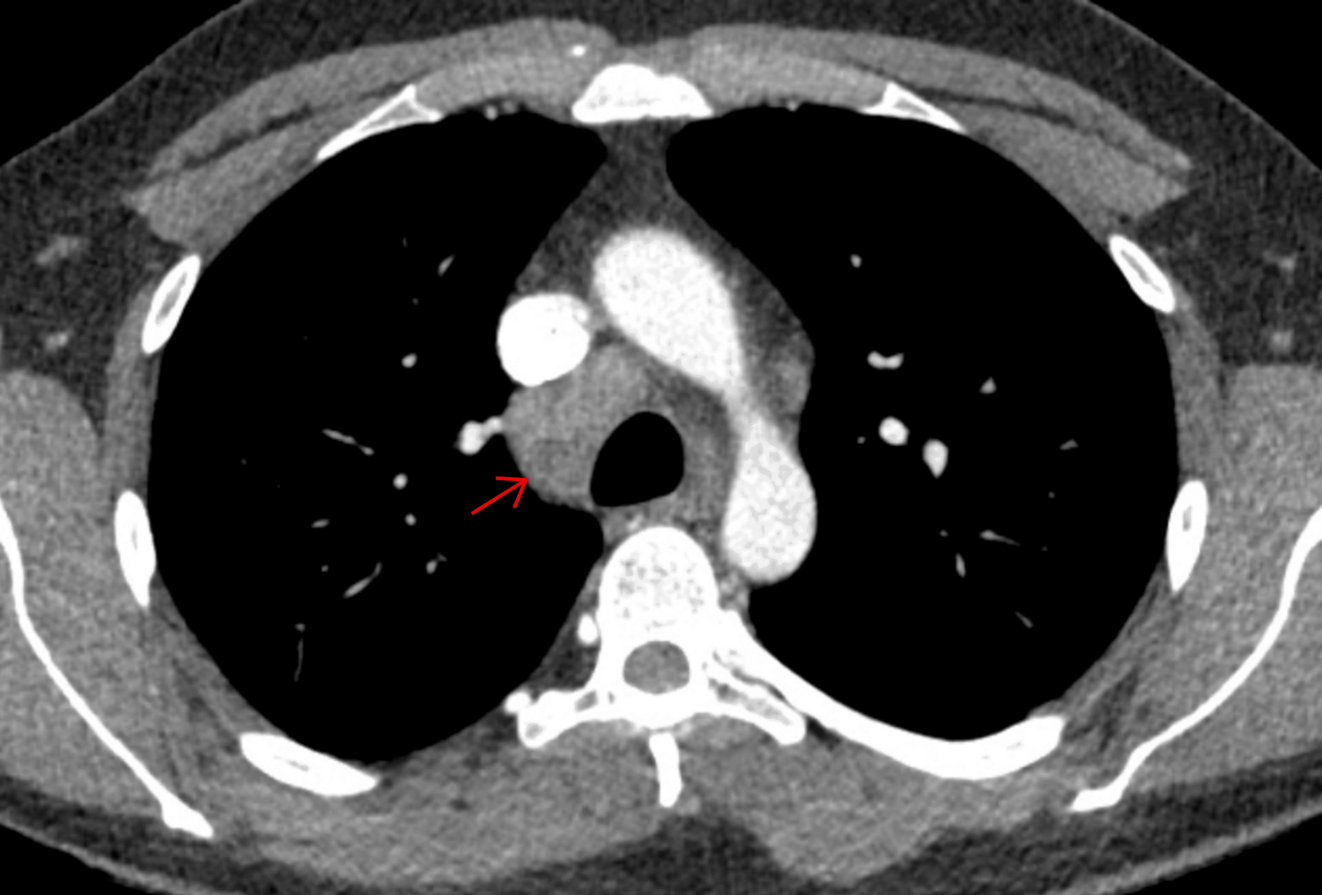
CT thorax showing enlarged right paratracheal lymph node CT: computed tomography
